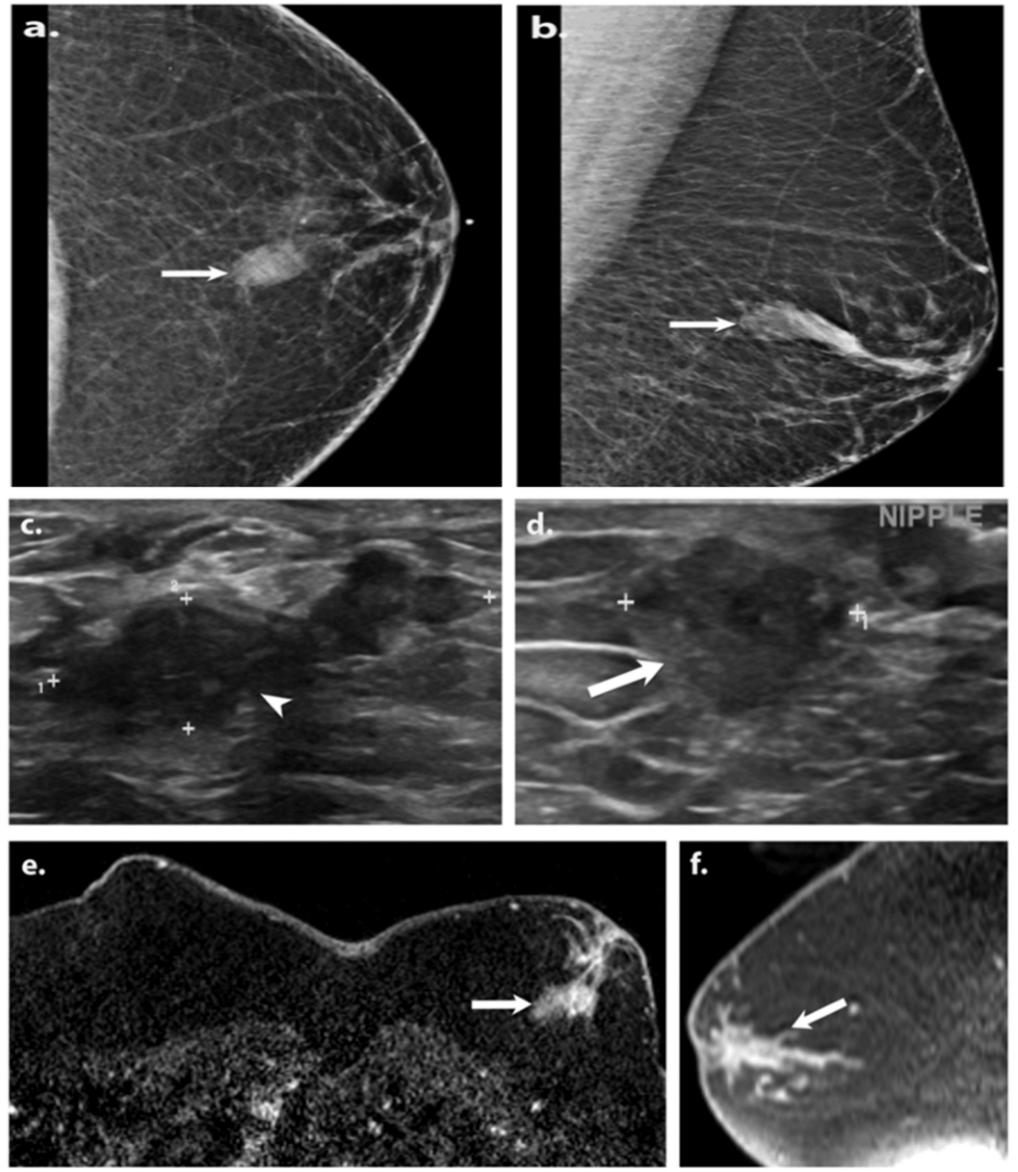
A 41-year-old African American male presented with intermittent left nipple discharge for one year. Cranio-caudal (CC) and mediolateral oblique (MLO) views of the diagnostic mammogram (a, b) show a tubular mass in the left retroareolar breast, likely representing a dilated duct (white arrows). Targeted greyscale ultrasound (c, d) images of the left retroareolar region at 11:00 o’clock demonstrates dilated duct (white arrowhead) with an irregular hypoechoic intraductal mass (white arrow). Left nipple punch biopsy revealed Paget disease of the breast and ductal carcinoma in situ, and subsequent ultrasound-guided biopsy showed invasive ductal carcinoma. Axial (e) and sagittal (f) postcontrast T1-weighted fat sat MRI images show an irregular heterogeneously enhancing mass in the left retroareolar breast (white arrows) involving the skin and nipple.
